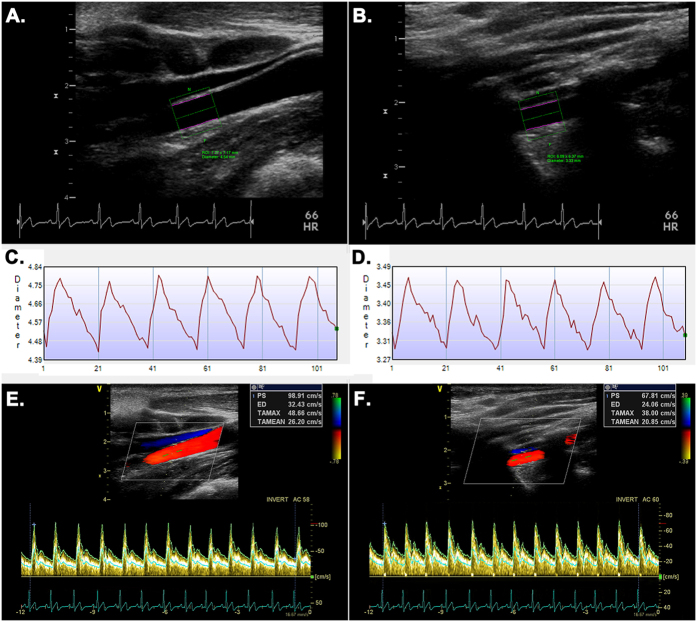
Measurement of cerebral blood flow at internal carotid (ICA) and vertebral arteries (VA) with color-coded duplex ultrasonography. The double purple lines within the region of interest (ROI, green rectangle) are the detected vessel inner walls in the high-resolution video clip of the ICA (A) or VA (B), which are used to track continuous pulsatile change of vessel diameter at the ICA (C) or VA (D) using an edge-detection and wall-tracking software (Brachial Analyzer, Medical Imaging Applications LLC, USA). Beat-by-beat recording of blood flow velocity at the site of vessel diameter measurement for the ICA (E) or VA (F) is presented. PSV, peak systolic velocity; ED, end-diastolic velocity; TAMAX, time-averaged max velocity; TAMEAN, time-averaged mean velocity.
